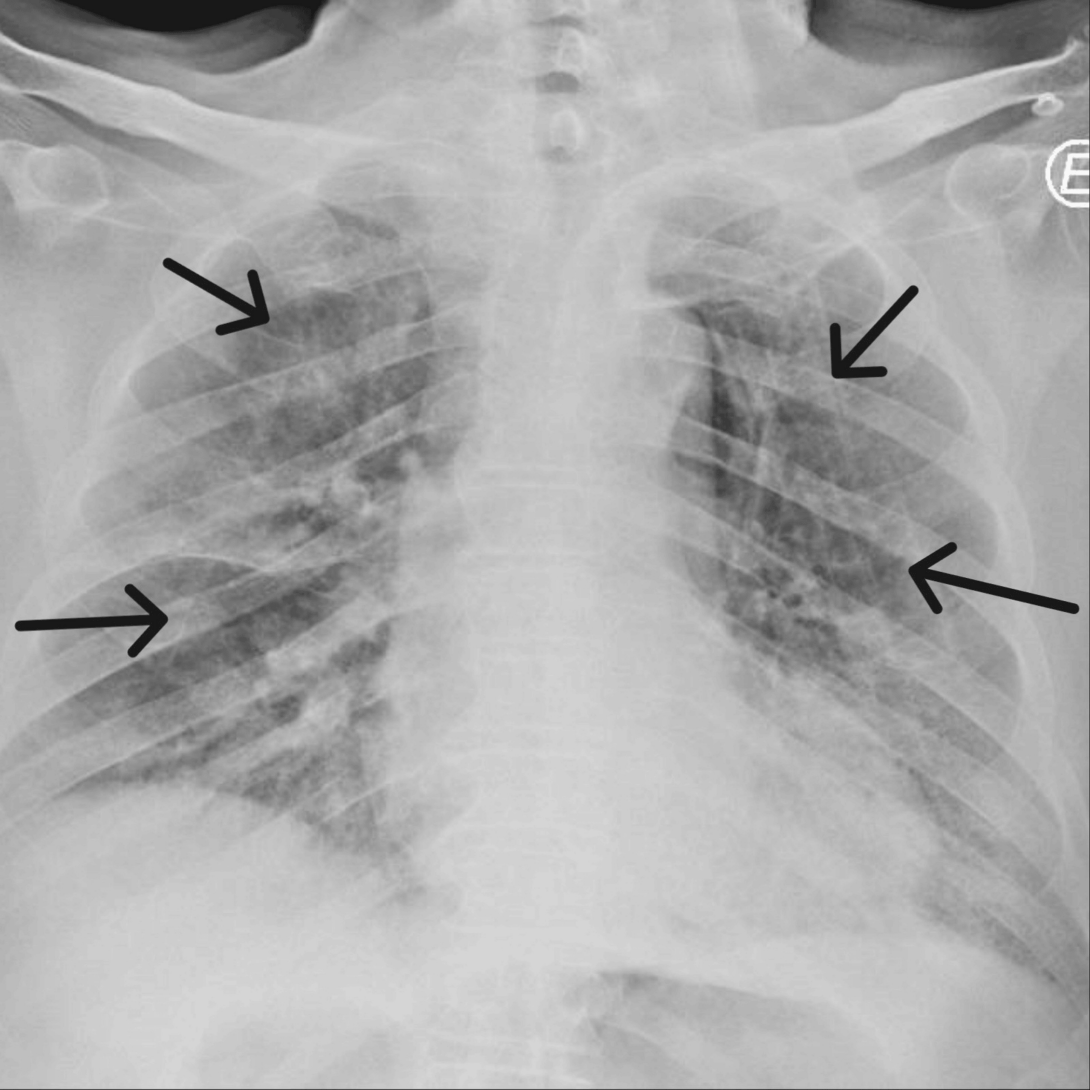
Chest X-ray upon admission showing bilateral infiltrates
